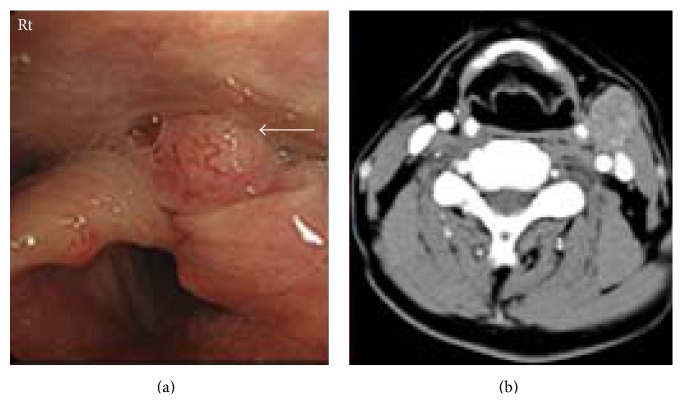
Contrast computed tomography of the neck showing a mass lesion in the left piriform recess, with metastasis to a lymph node on the left.
